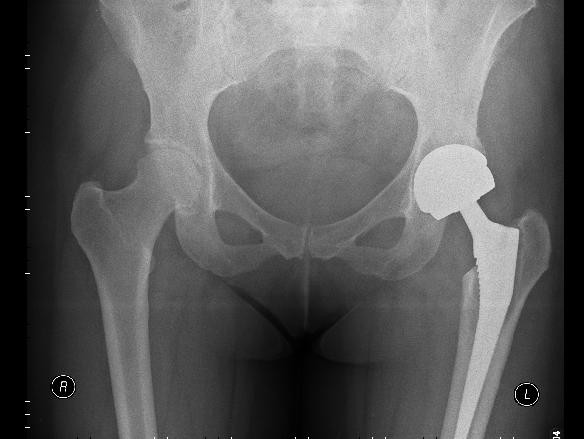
Radiograph showing a LHM replacement.
